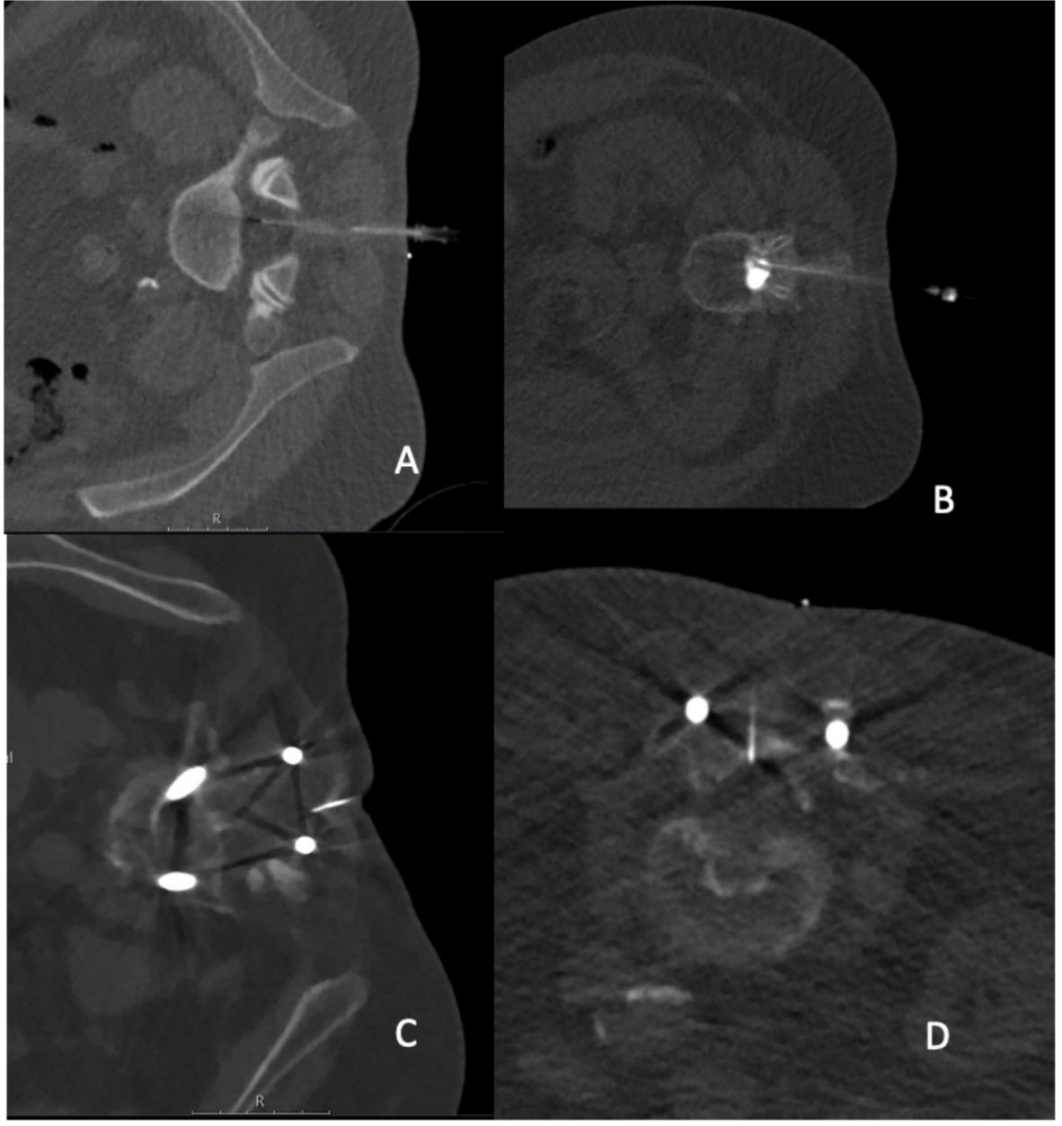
Examples for image-guided punctures at the lumbar spine for administration of intradural contrast agent prior to computed tomography (CT) myelography in different patients performed with a low-dose (LD) protocol for scanning, with resulting CT scans rated as perfect (A; 120 kV; 30 mA; CTDI_vol_ 1.2 mGy × 12; DLP 14.4 mGy*cm), good (B; 120 kV; 30 mA; CTDI_vol_ 1.2 mGy × 1; DLP 1.2 mGy*cm), medium (C; 140 kV; 27 mA; CTDI_vol_ 1.2 mGy × 1; DLP 1.2 mGy*cm), and poor (D; 120 kV; 30 mA; CTDI_vol_ 2.4 mGy × 9; DLP 21.6 mGy*cm) regarding overall image quality (A–D: bone kernel; windowing L = 750, W = 2500, slice thickness: 3.33 mm, iDose4).
